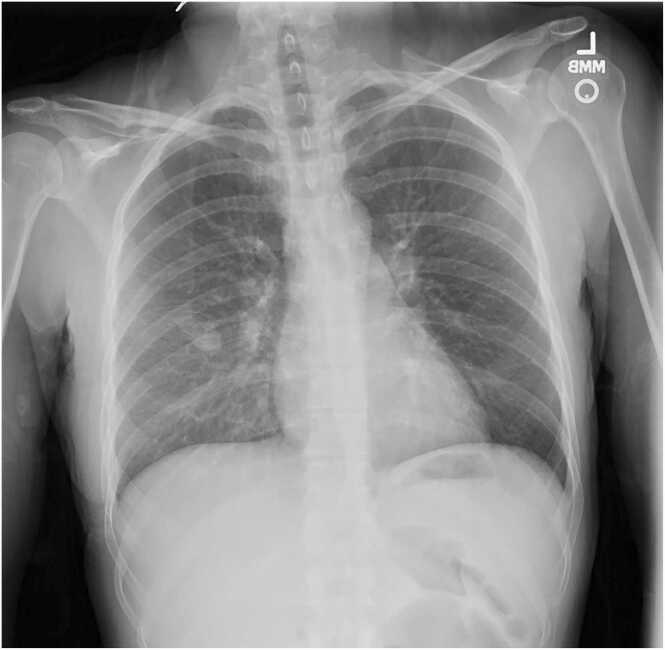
Initial chest xray image with mild right perihilar infiltrate and right middle lobe lung nodule.
